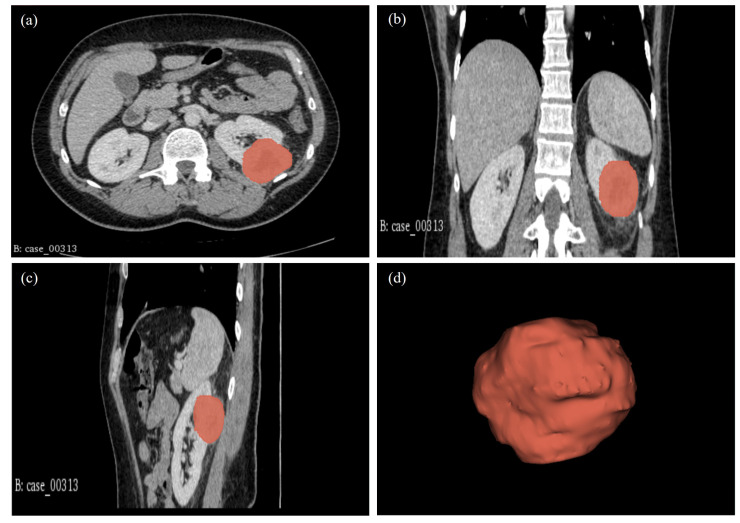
Manual segmentation of the 3D image slices using the 3D Slicer software: version 4.11.20210226. (a) CT scan axial plane; (b) Coronal plane; (c) Sagittal plane; and (d) 3D VOI.
